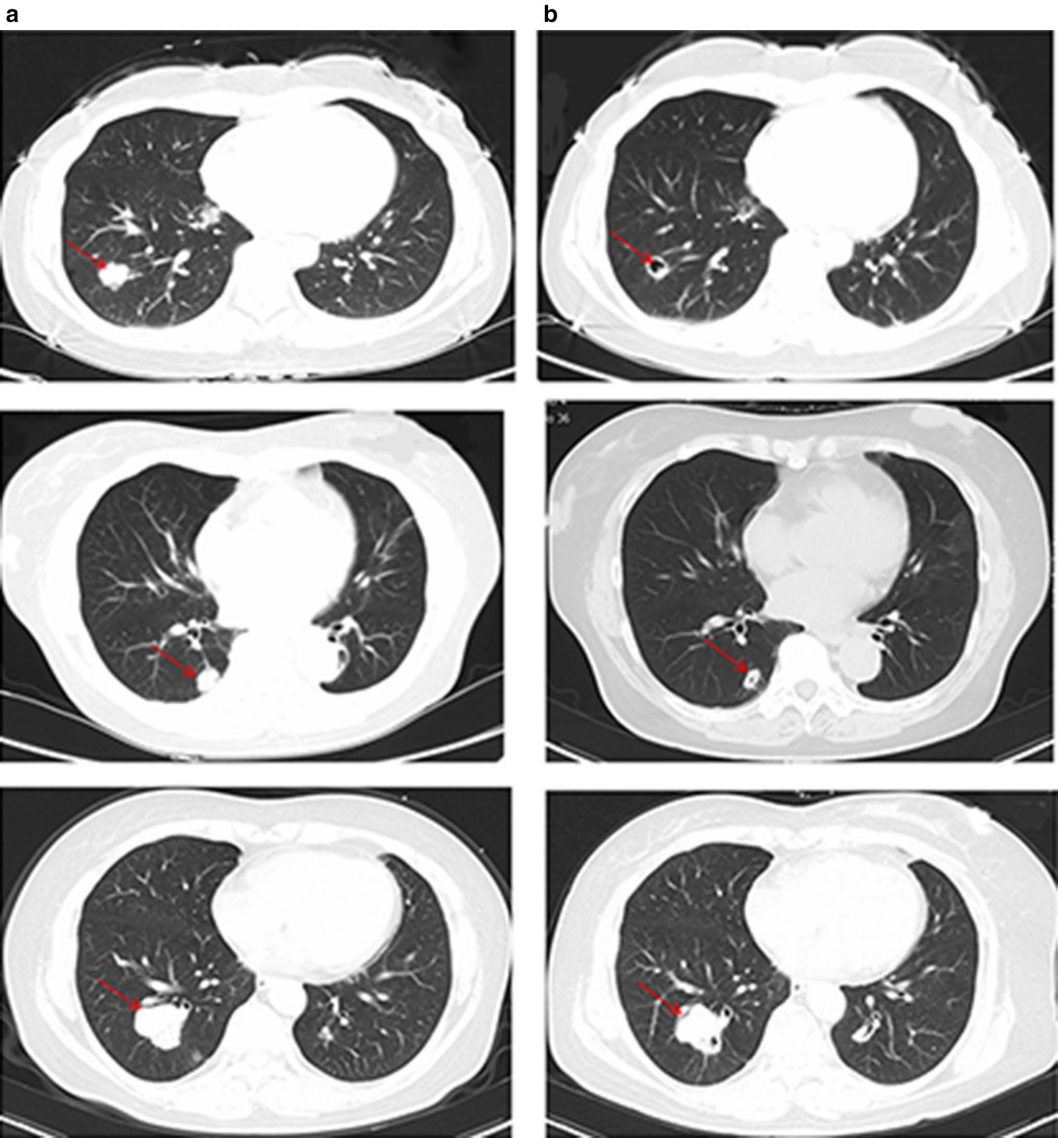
Baseline (a) and after therapy (b) CT displaying the onset of cavitation in three patients treated with bevacizumab. The arrow highlights tumor metastases with cavitation
